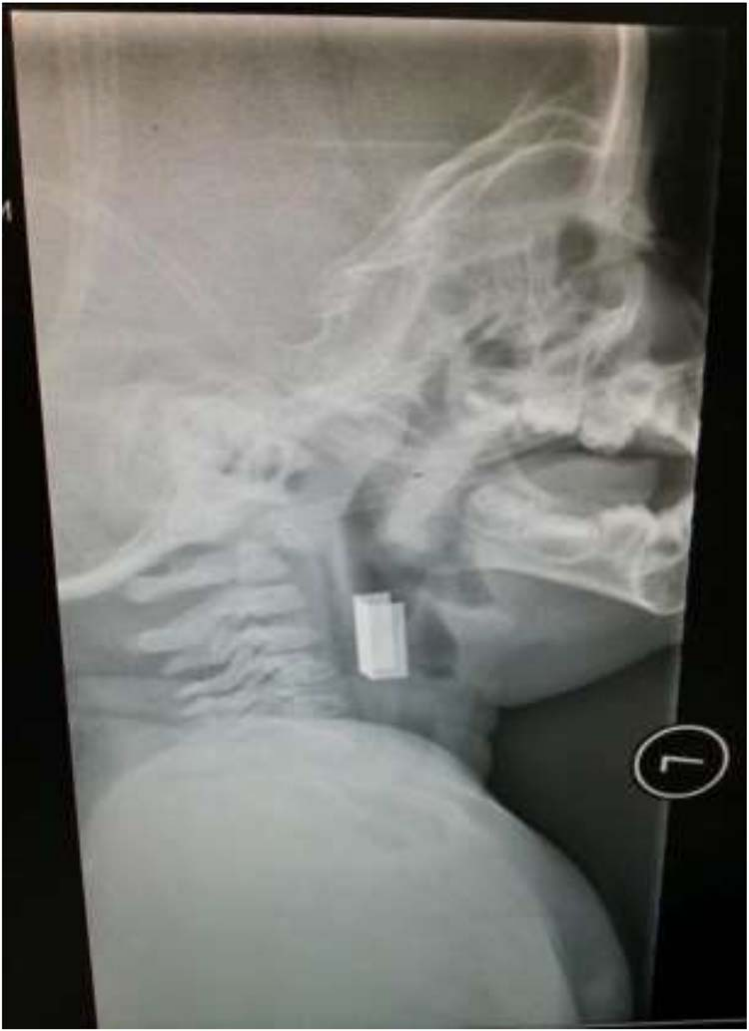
Lateral neck X-ray showing a radio-opaque object in the upper airway.
